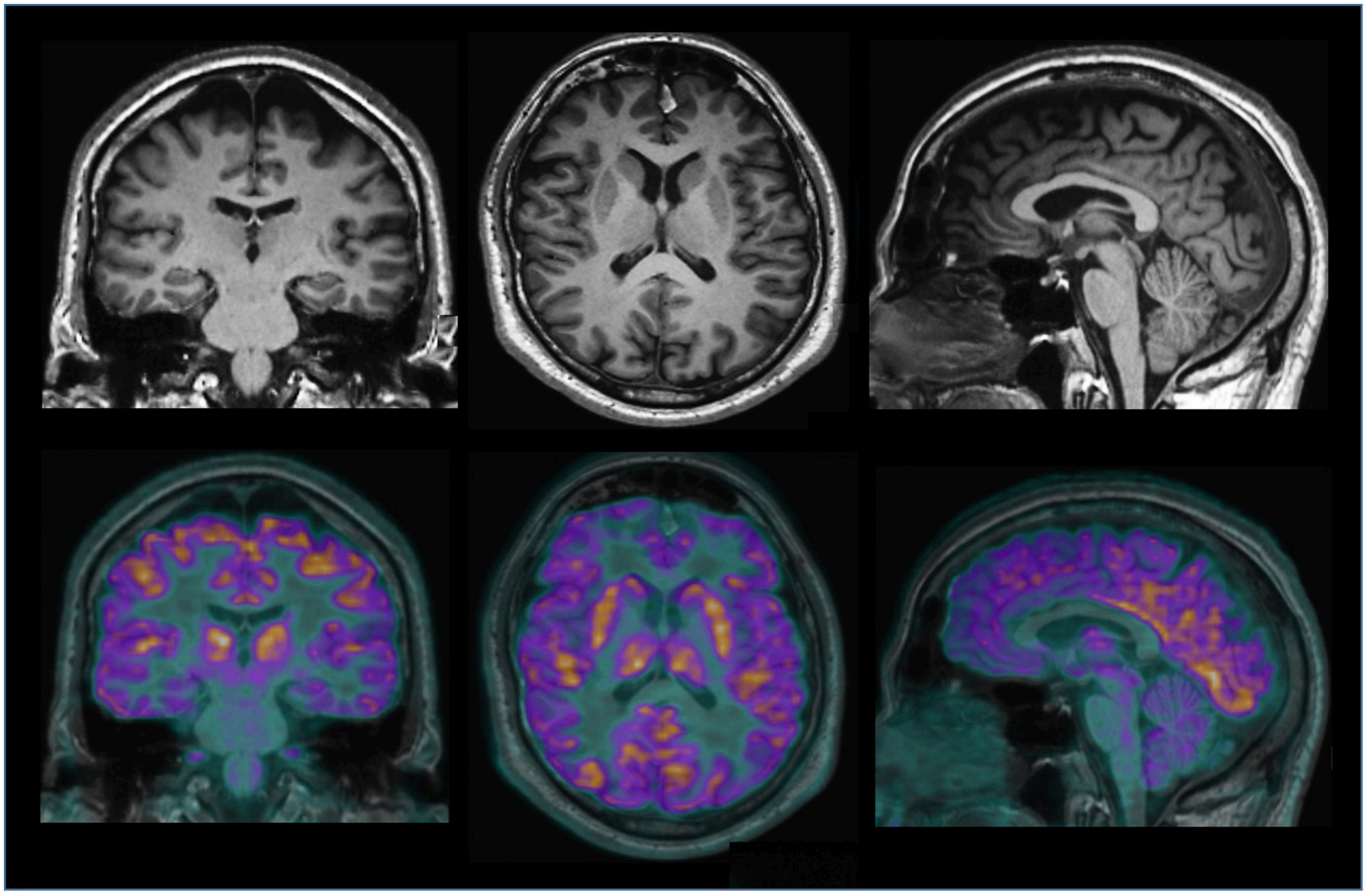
Hybrid brain PET/MRI imaging (Biograph mMR, Siemens) in a normal subject. The top picture shows anatomical MRI 3D T1-weighted images on the coronal, axial and sagittal slices. In the figure above, a metabolic 18FDG PET image of the same individual is combined with the MRI image to obtain information on the morphology, anatomy and metabolism of the brain.
